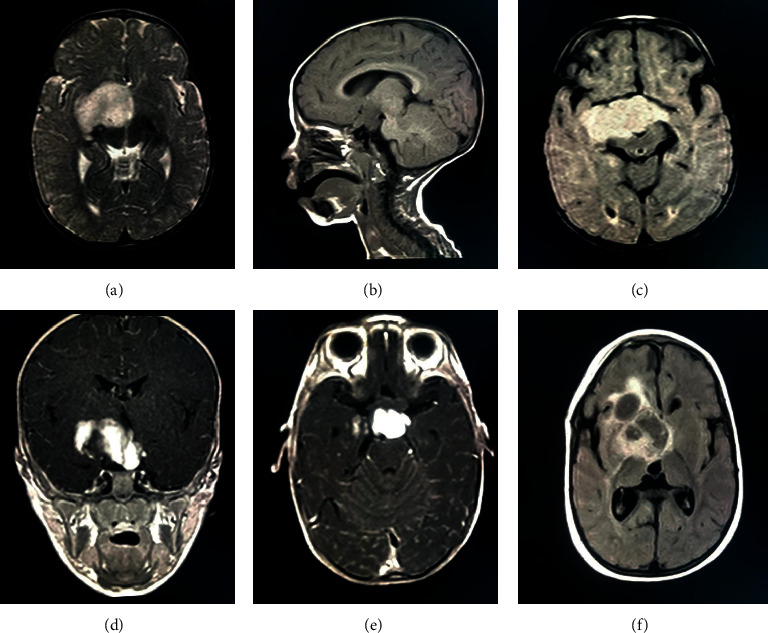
Preoperative axial T2-weighted MRI (a), sagittal T1-weighted MRI (b), and axial FLAIR MRI (c) elucidate a suprasellar lesion isointense on T1-weighted MRI (b) and hyperintense on T2-weighted MRI (a) homogeneously enhanced upon contrast administration (d); 6-month postoperative MRI (e) shows a residual mass with the cystic component (f).
